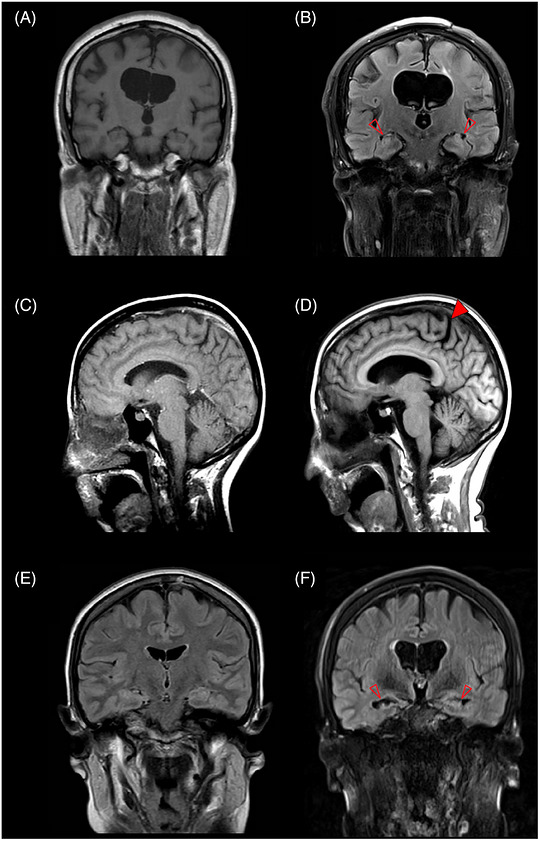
Examples of neuroimaging changes in reported patients. (A and B) Patient 1. Sequential images (A, T1‐weighted; B, T2‐weighted fluid‐attenuated inversion recovery [FLAIR], 4 years later) show progressive hippocampal atrophy (empty red arrowheads). (C and D) Patient 5. FLAIR images 18 months apart show progression of parietal atrophy, most apparent in the pars marginalis (red arrow). These changes are contralateral to the hematoma and therefore unlikely to relate to this. (E and F) Patient 9. At presentation, there is no evidence of regional or generalized atrophy on coronal FLAIR images (E). Repeat imaging 2 years later (F) shows extensive atrophy, particularly affecting the hippocampi and medial temporal lobes (empty red arrowheads).
